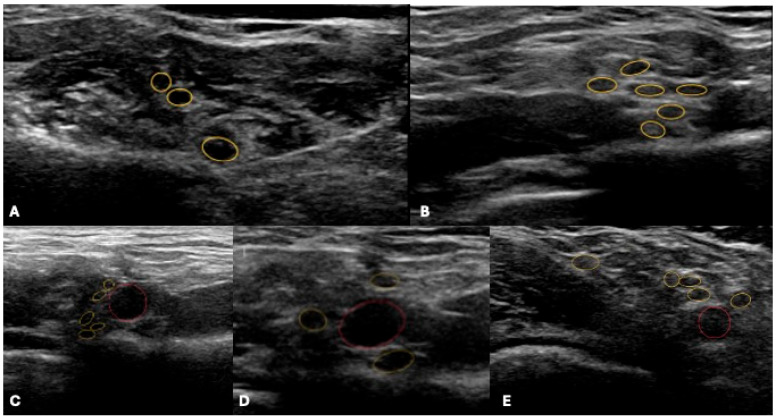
Ultrasound depiction of the brachial plexus at different anatomical levels. (A) Trunks (7u, lower) within the interscalene gap. (B) Divisions in the typical “bunch-of-grapes” configuration and (C) in the costoclavicular space. (D) Cords (posterior, medial, lateral) arranged around the subclavian artery (in red) in the retropectoralis minor space. (E) Terminal branches in the axillary projection: musculocutaneous (MCN), axillary (AN), radial (RN), median (MN), and ulnar (UN). Nerve structures are highlighted in yellow, and the subclavian artery in red.
